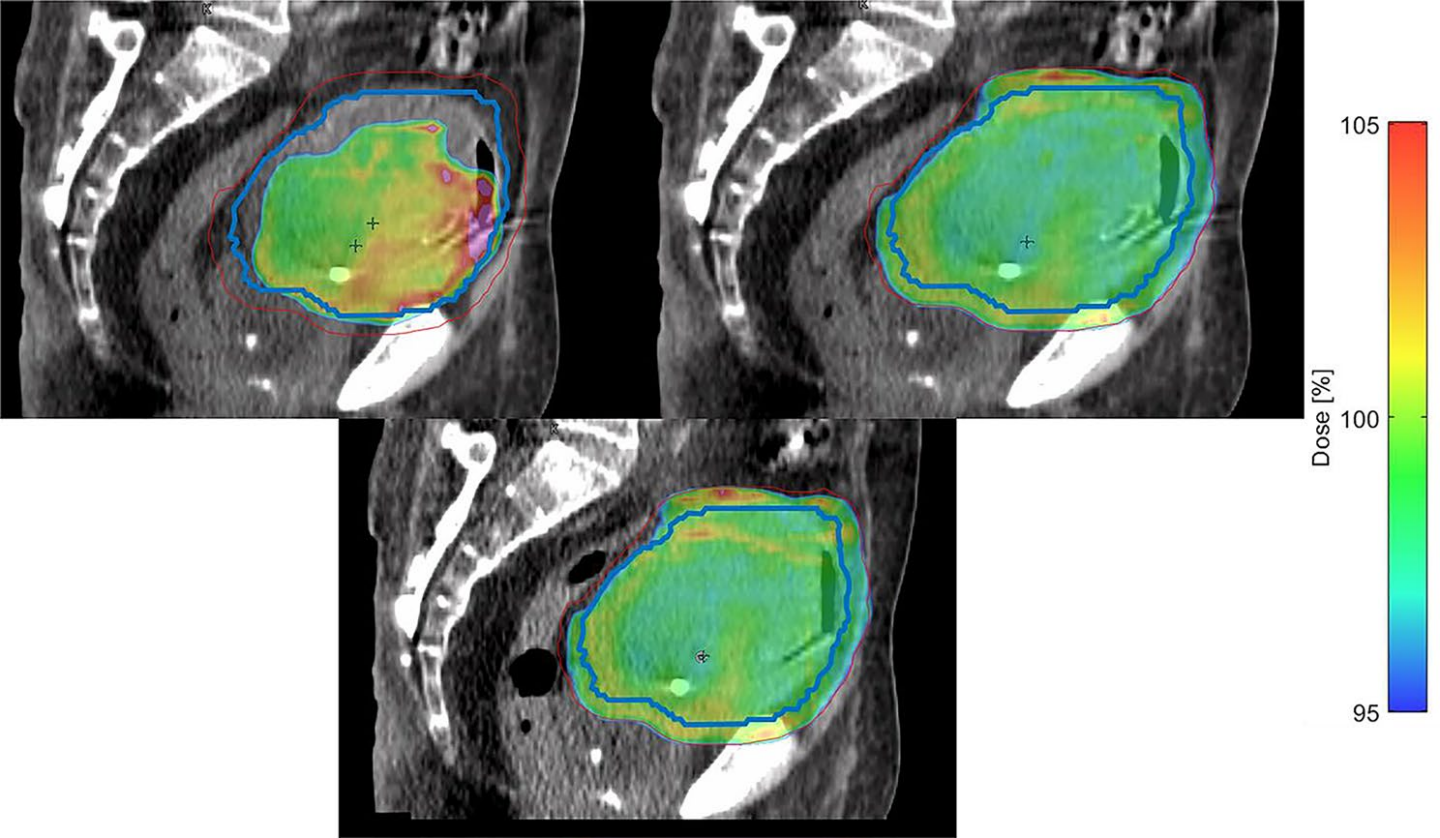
Visualization of the 95% isodose (blue) with a color wash up to 105% (red) of the prescribed dose. The scheduled plan (SCH, top-left) shows the CTV (blue) with insufficient coverage, likely due to bladder volume fluctuations. In contrast, the adapted plan (ADP, top-right) demonstrates an optimized dose distribution with improved target coverage. Bottom: Dose distribution reconstructed on the post-adaptive vCBCT (VER). These images show patient 8, fraction 7
